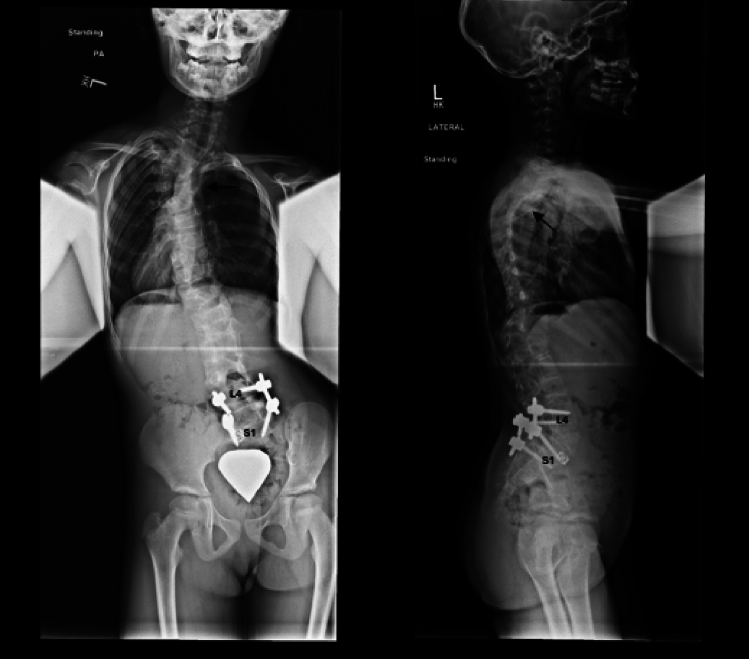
Case 2 Pre-operative PA and lateral X-ray of a 9-year-old female with congenital scoliosis
